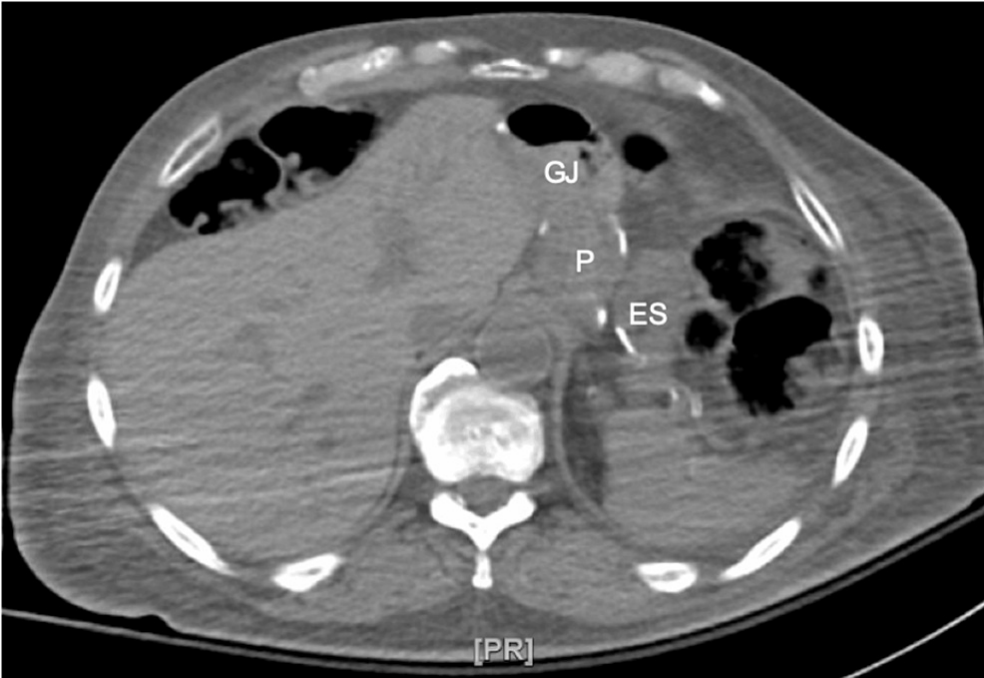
Axial CT scan of the abdomen showing a gastric pouch (P) laterally separated from the excluded stomach (ES), with a gastrojejunal (GJ) anastomosis anteriorly connected to the gastric pouch CT: computed tomography, P: gastric pouch, ES: excluded stomach, GJ: gastrojejunal
